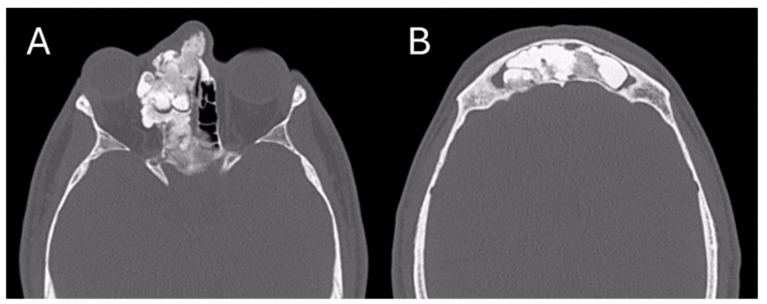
Pre-operative CT image of Patient 2. Two axial cuts are appreciated (A) The lesion localized at the ethmoidal level (B) The lesion localizedin the right frontal sinus.
